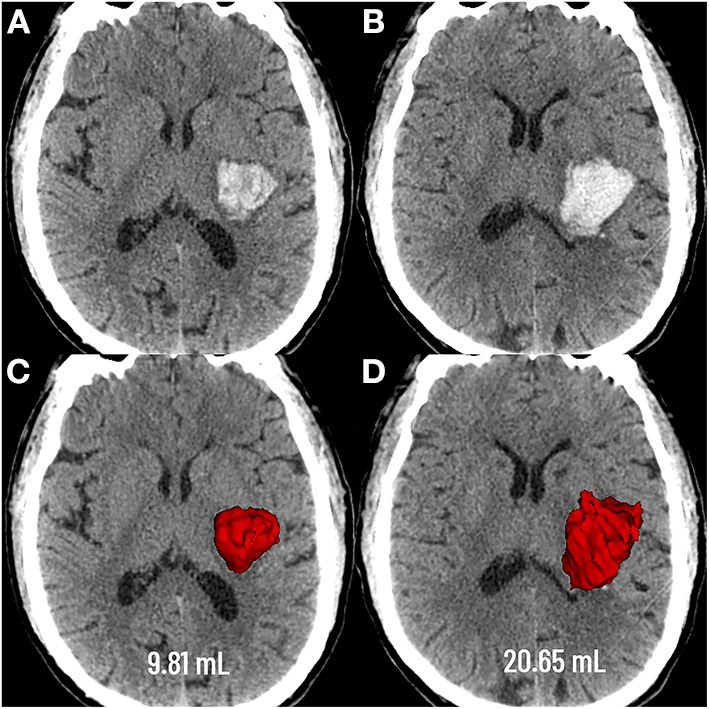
Graphic representation of the extent of deep hematoma involvement on non-contrast CT. Deep hematoma involving the PLIC was seen as early as 3.4 h following neurological deficits (A), with progressive expansion over the subsequent 23.1 h (B). Baseline CT showed a hematoma volume of 9.81 mL (C), whereas the volume was 20.65 mL at the follow-up CT (D). PLIC, posterior limb of the internal capsule.
